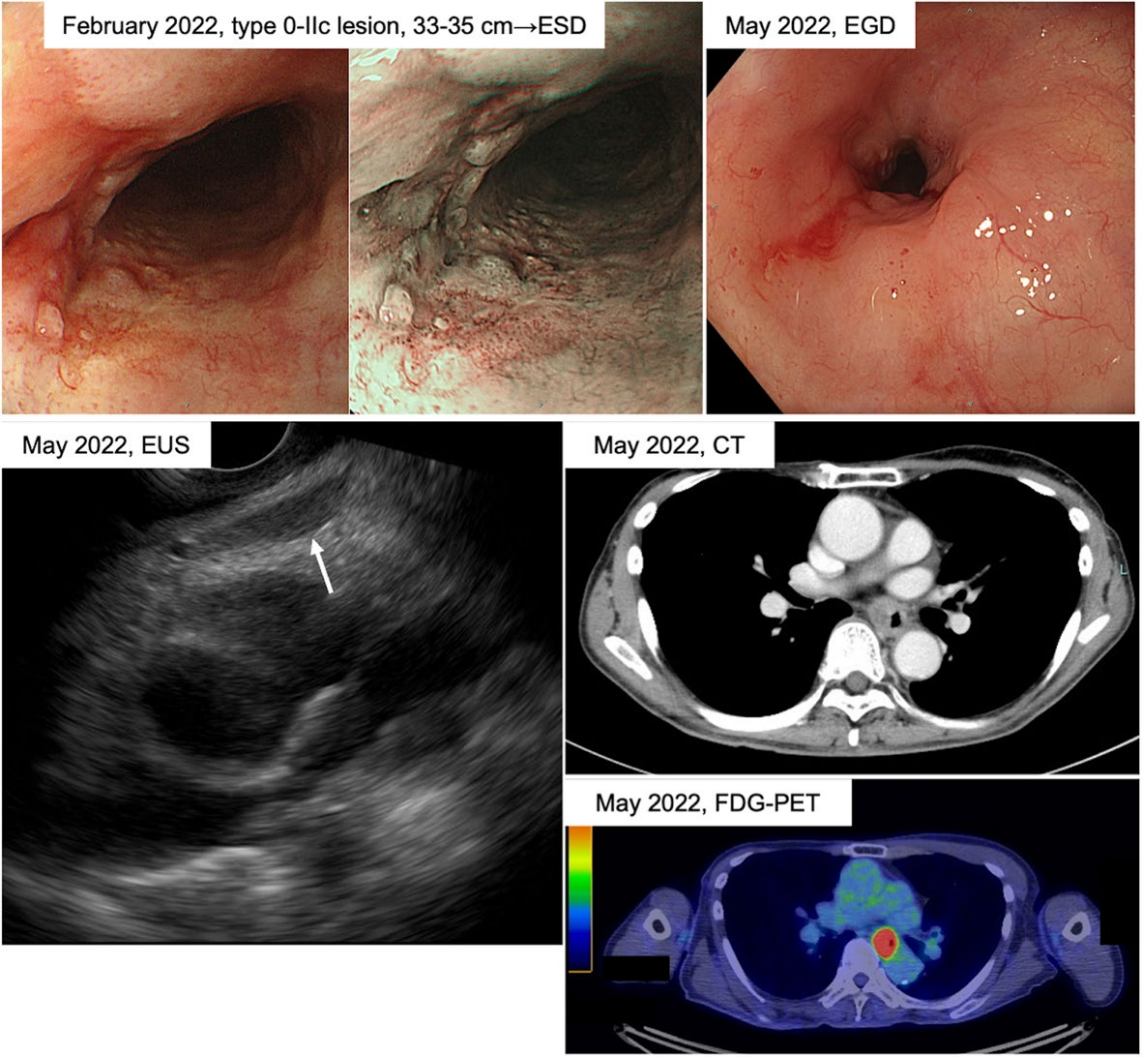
Endoscopic, CT, and FDG-PET findings before surgery. In February 2022, upper gastrointestinal endoscopy detected a type 0–2c lesion located 33–35 cm from the incisors, and ESD was performed for removal of this lesion. In May 2022, EGD and EUS detected stenosis attributable to intramural tumor recurrence in the previously treated area. CT and FDG-PET imaging detected neither LN nor distant metastases. ESD, endoscopic submucosal dissection; EGD, esophagogastroduodenoscopy; EUS, endoscopic ultrasound sonography; CT, computed tomography; FDG-PET, 18F-fluorodeoxyglucose positron emission tomography
